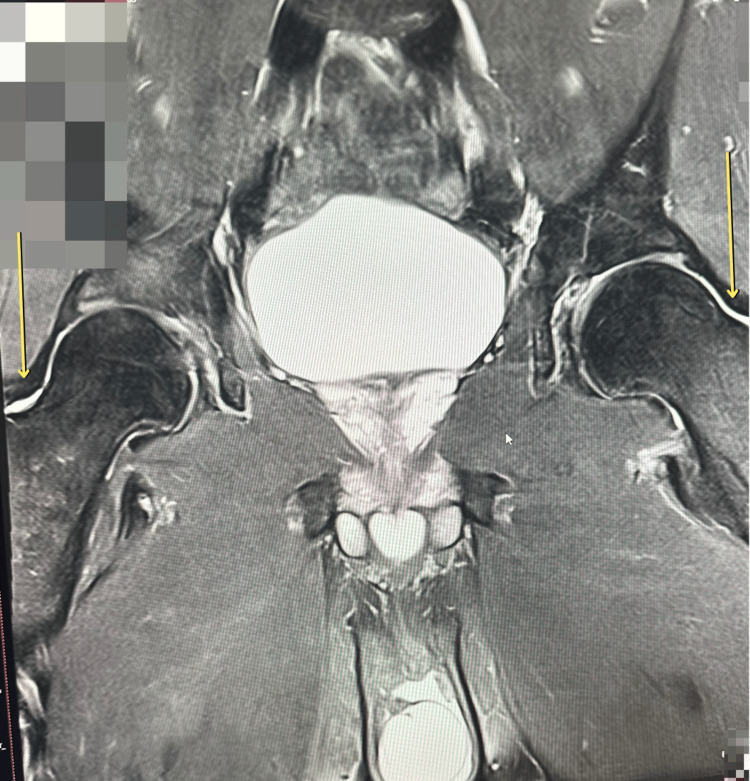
Coronal pelvic MRI (T2 fat-saturated/STIR sequences), demonstrating capsular hypertrophy (see yellow arrows) MRI: magnetic resonance imaging; STIR: short-tau inversion recovery
